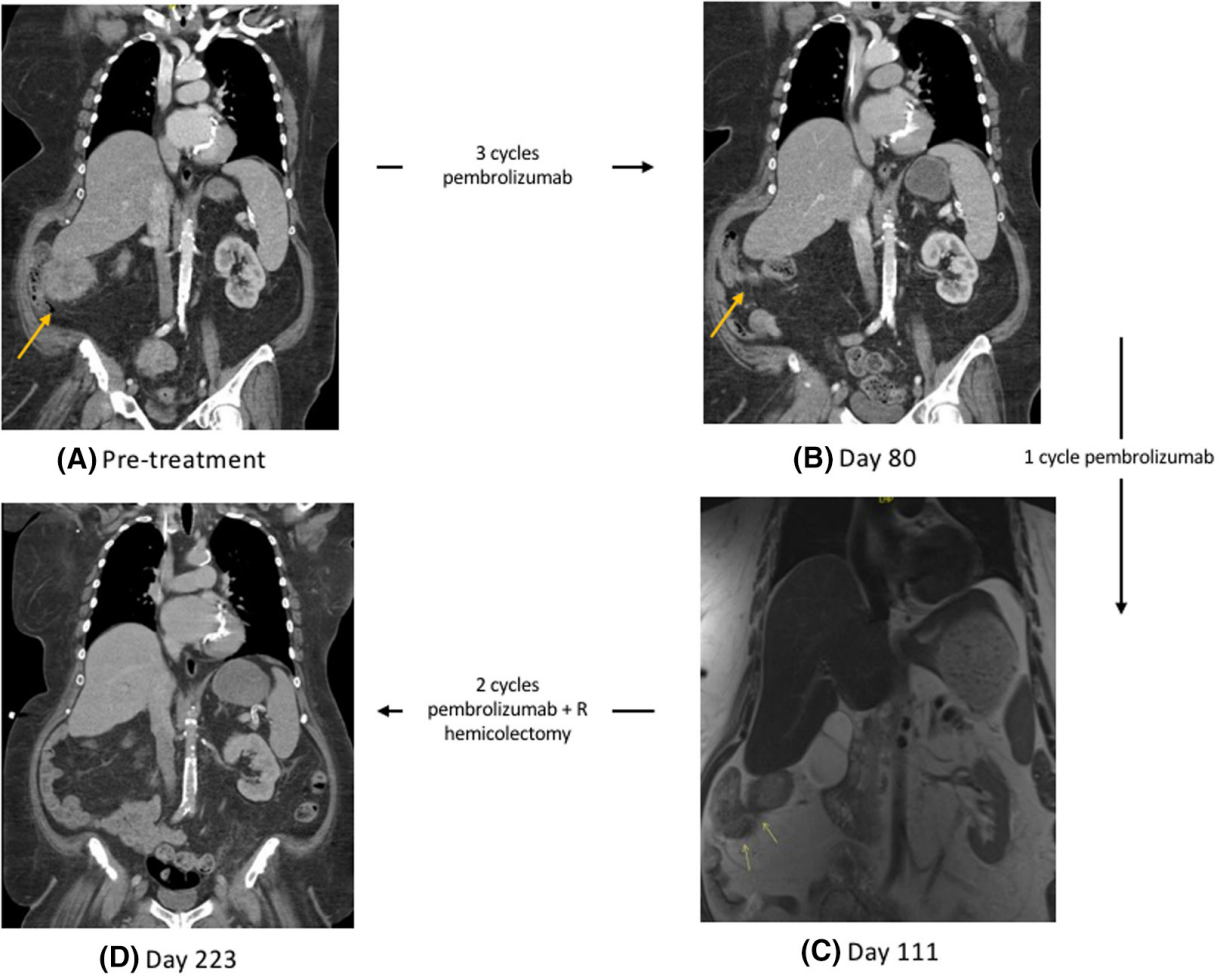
Baseline contrast enhanced CT showing circumferential soft tissue mass contiguous to the right liver inferior margin with question tumor invasion to the liver capsule (A). Follow up contrast enhanced CT following 3 cycles of pembrolizumab and follow up MRI following another 3 cycles of pembrolizumab showing interval decrease in cecal mass without definite involvement with the inferior right hepatic lobe (B, C). MRI post hemicolectomy and pembrolizumab showing post‐surgical hemicolectomy (D).
